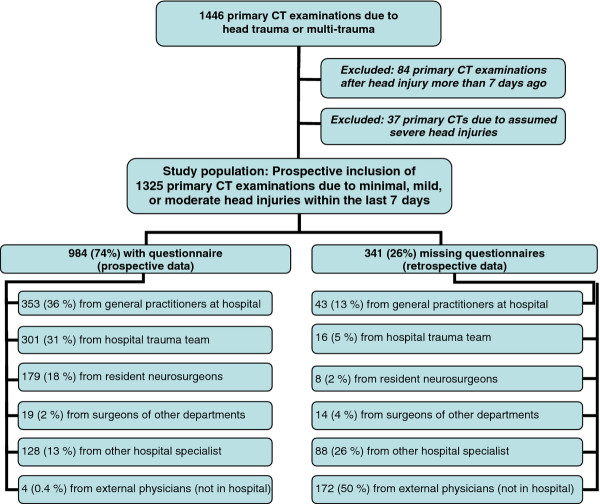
The inclusion and exclusion process. The inclusion and exclusion process leading to a study population of 1325 primary CT examinations after recent minimal, mild, or moderate head trauma is shown.
